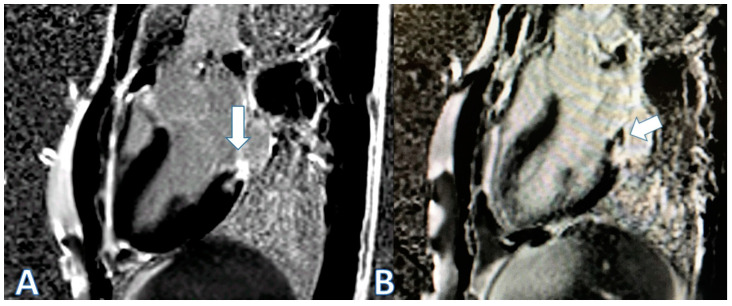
Cardiac MRI images. (A) Three-chamber view. Enhancement of both pericardial leaves and focal subepicardial LGE on basal inferolateral, lateral, and inferior walls (white arrow), with preserved biventricular function. (B) Three-chamber view, 3 months: persistence of focal LGE on basal inferolateral wall (white arrow).
